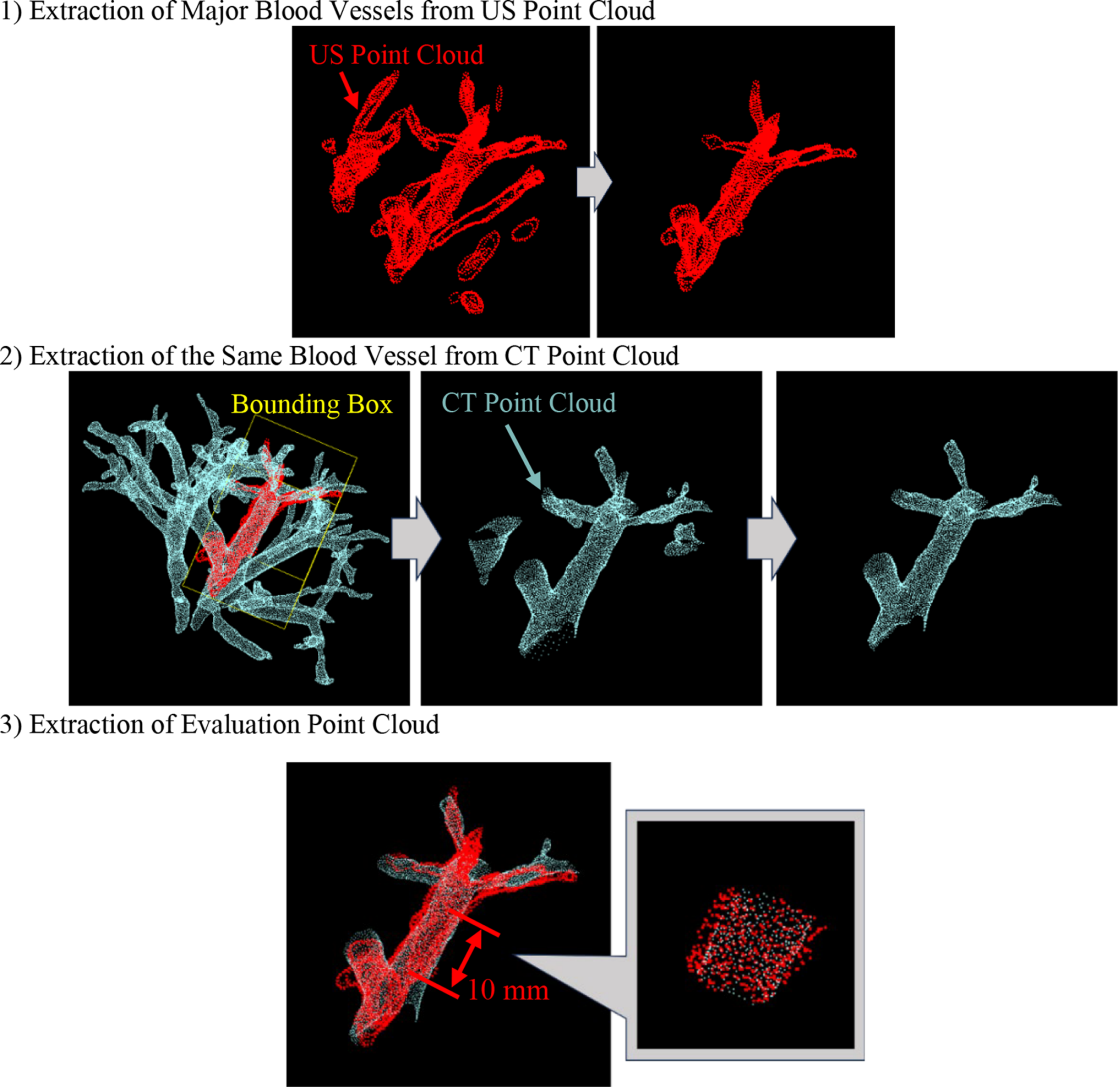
Analysis procedure of the 3D US–CT fusion results
